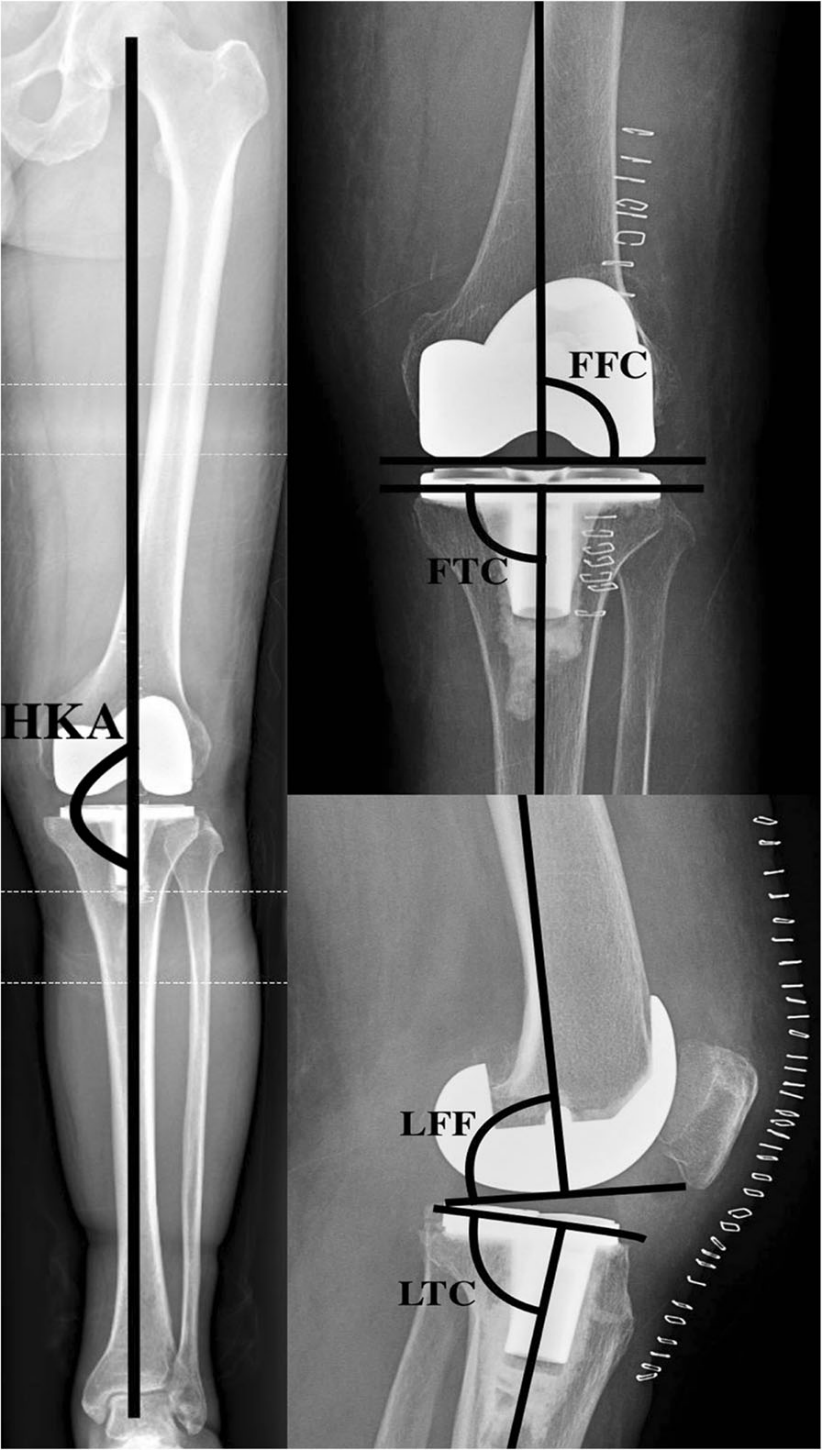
Antero/posterior and sagittal radiographs showing the standard graphic patterns of HKA, FFC, FTC, LFF, and LTC. HKA, hip-knee-ankle angle; FFC, frontal femoral component angle; FTC, frontal tibial component angle; LFF, lateral femoral flexion angle; LTC, lateral tibial component angle
